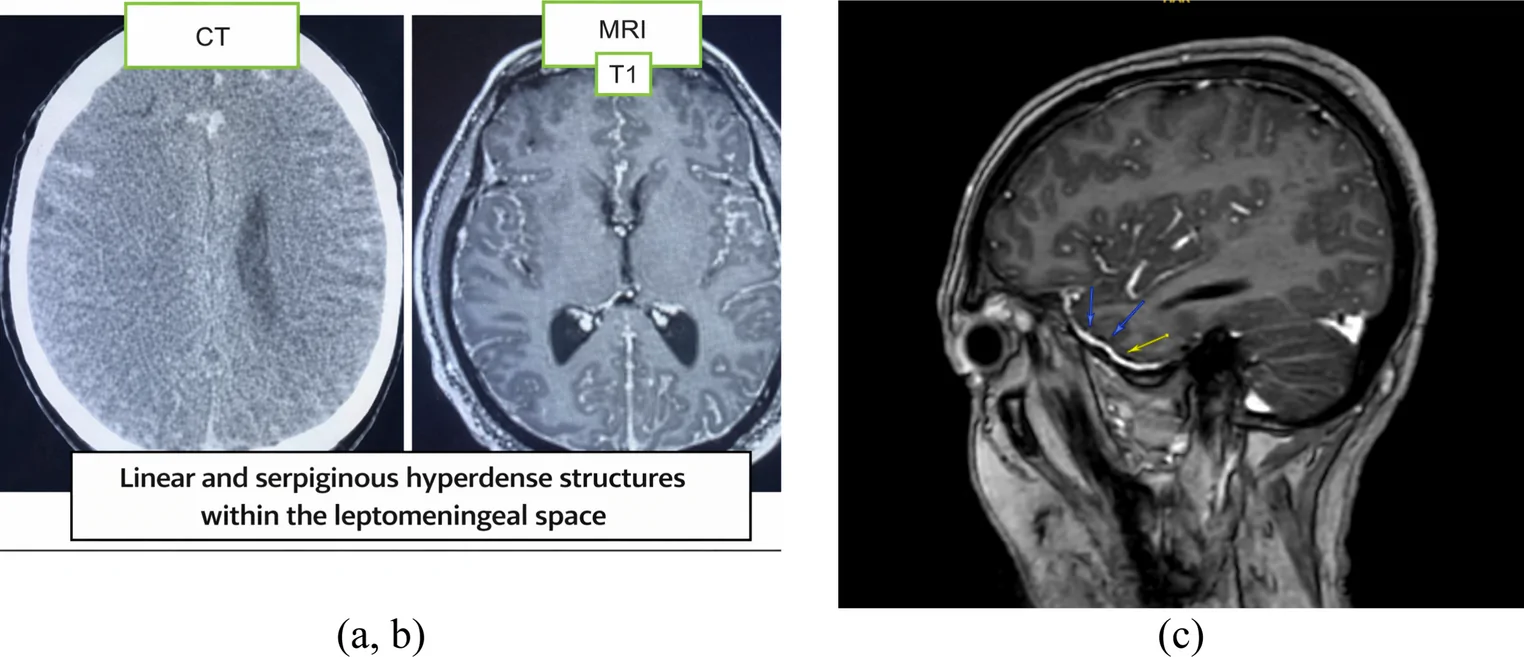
Neuroimaging findings. a Non-contrast CT showing diffuse linear and serpiginous leptomeningeal hyperdensities consistent with calcified amyloid deposits, with superimposed focal cortical hyperdensities compatible with subarachnoid hemorrhage. b, c Contrast-enhanced T1-weighted MRI demonstrating irregular leptomeningeal and pachymeningeal thickening and enhancement, predominantly involving cortical and subarachnoid regions
